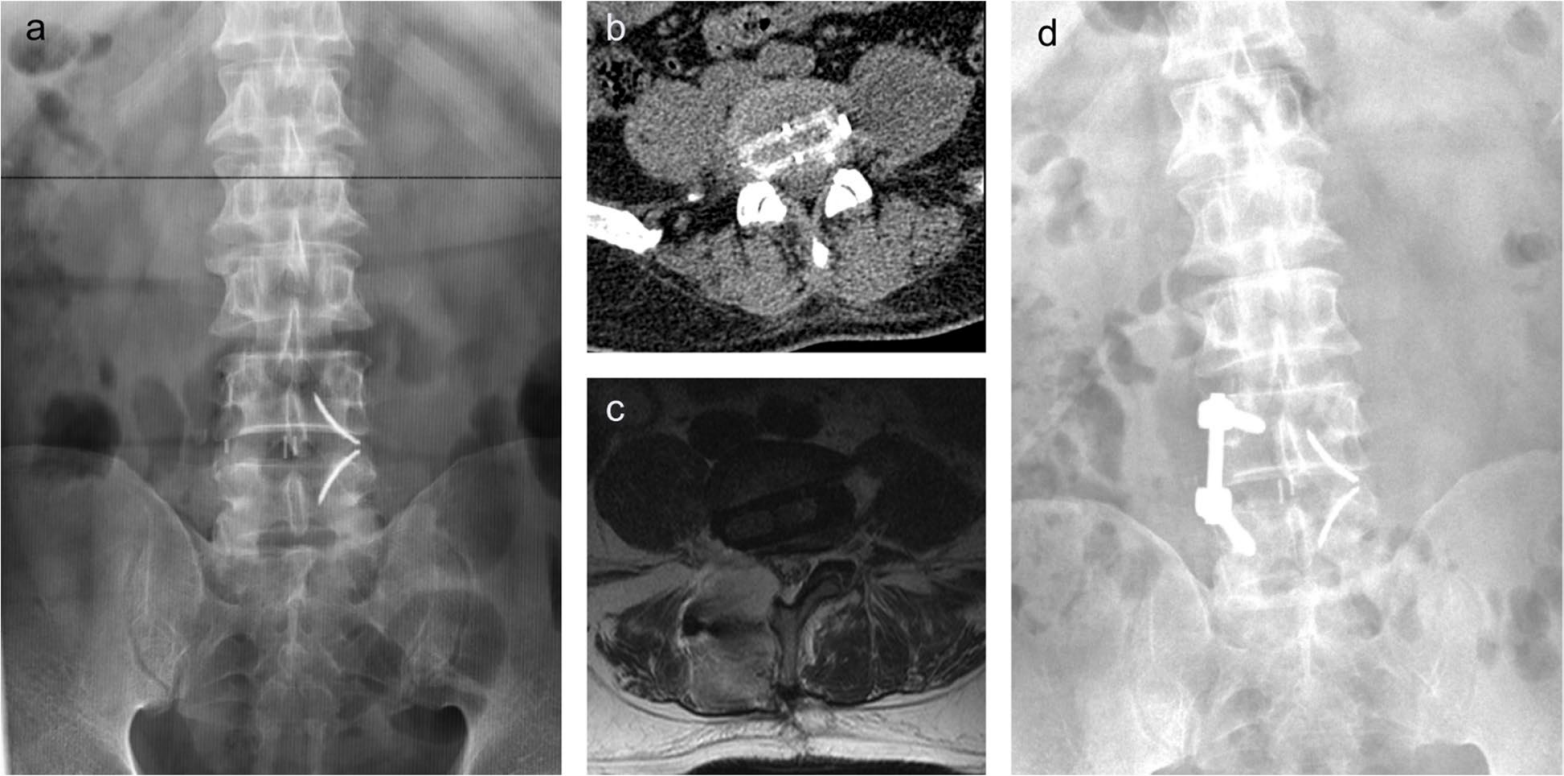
A case was revised with TLIF for contralateral nerve root injury. a Anteroposterior X-ray of lumbar spine after XLIF. b Axial CT showed that the right nerve root was compressed by the cage after XLIF. c Axial T2-weighted MRI image after revision indicated right decompression. d Anteroposterior X-ray of lumbar spine after revision
